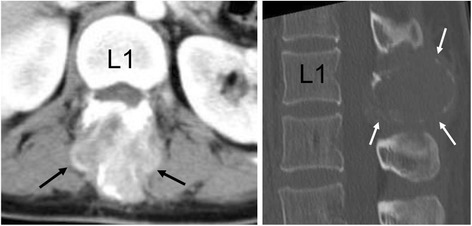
Preoperative computed tomography (CT). *Left*: Axial contrast-enhanced CT image reveals a heterogeneously enhanced mass (*arrows*) in the L1 vertebra. *Right*: Sagittal reconstructed bone window CT image of the lumbar spine shows an expansile lytic lesion (*arrows*) in the posterior L1 vertebral arch. Abbreviation: *L1* body of the first lumbar vertebra
